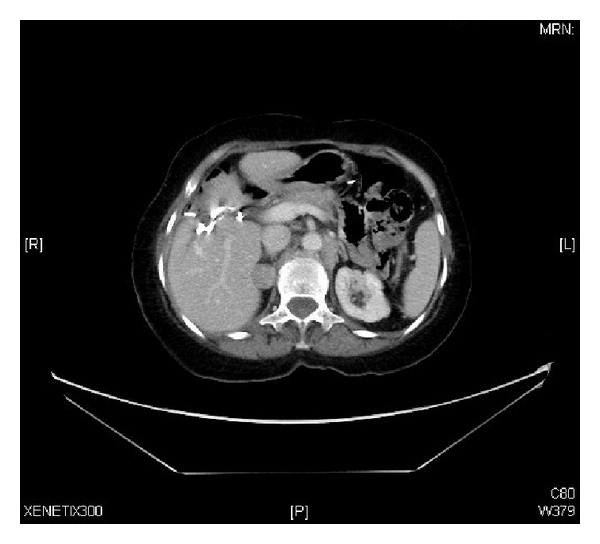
Follow-up abdominal CT at one year showing the recurrent soft tissue mass adjacent to the liver inseparable from the right adrenal and interval increased in size of the left para-aortic lymph nodes.
